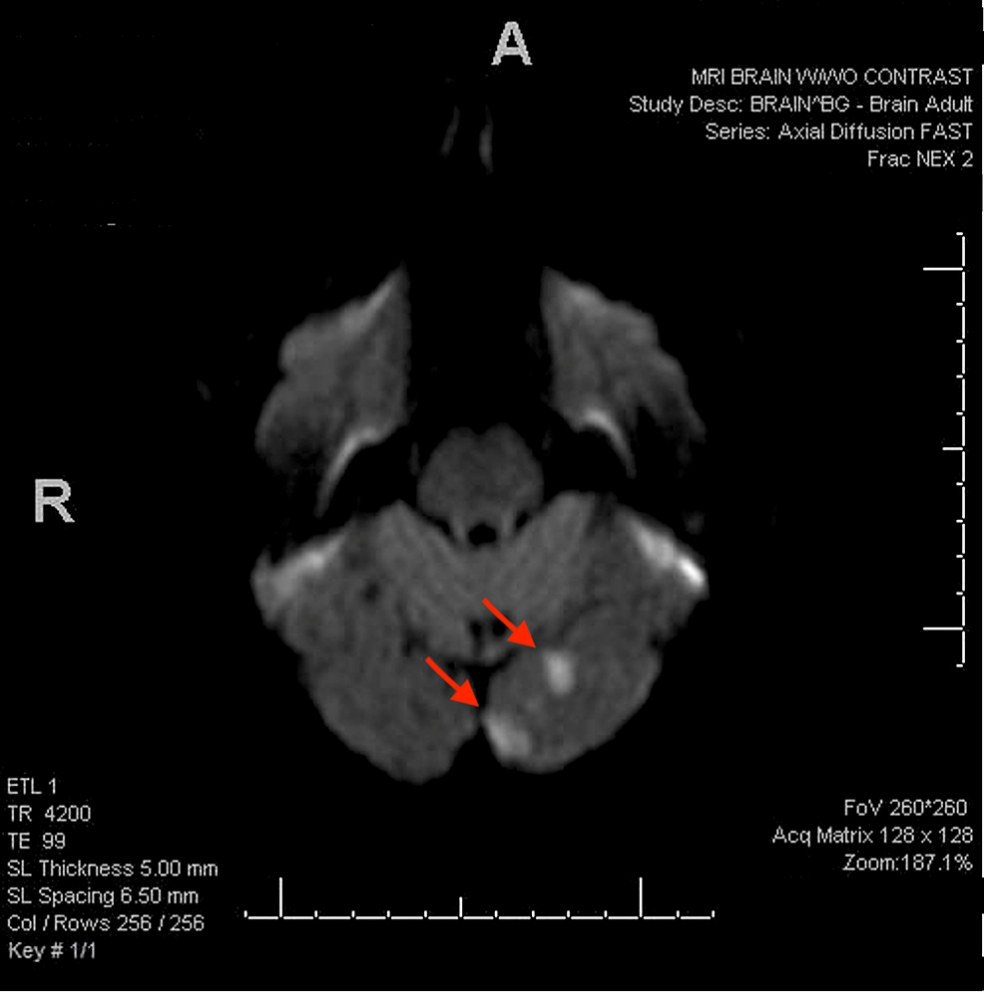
MRI of the brain demonstrating multifocal acute infarcts in the left occipital lobe. MRI: magnetic resonance imaging
